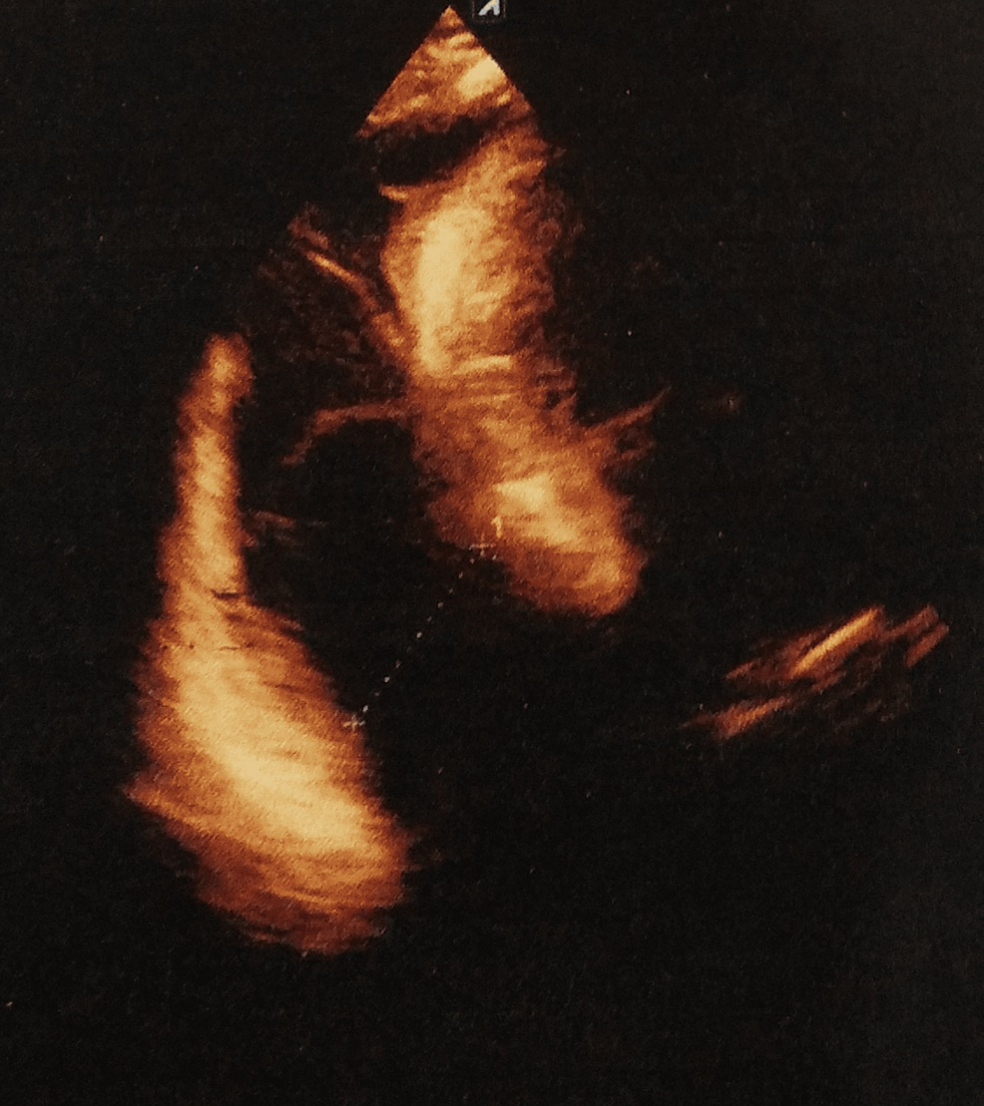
Color Doppler echocardiography showing massive pericardial effusion
